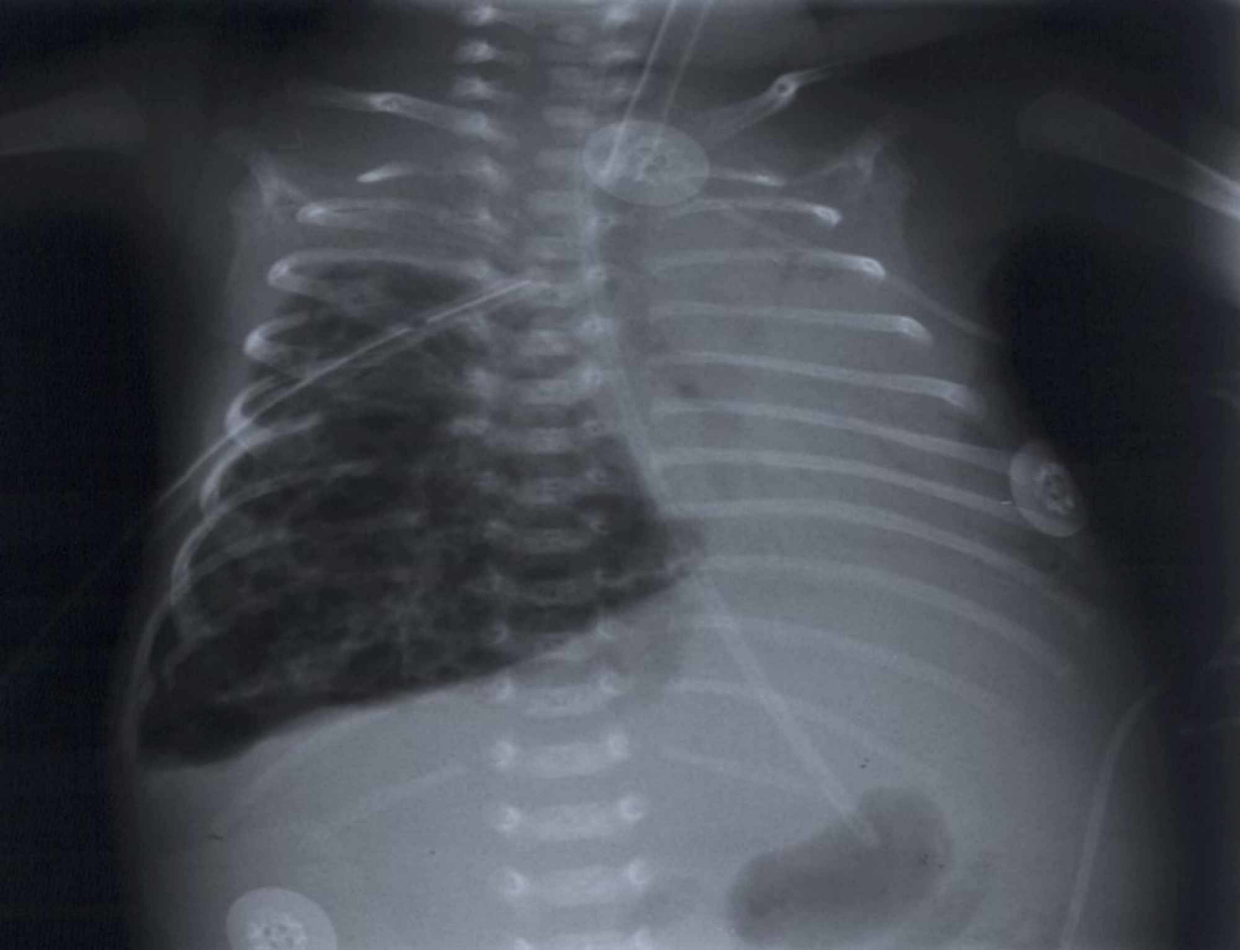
Chest radiograph, posterior-anterior view, performed four days after birth, showing hyperinflation of the right middle lobe. The mediastinal shift to the left and atelectasis of the other lobes are present
